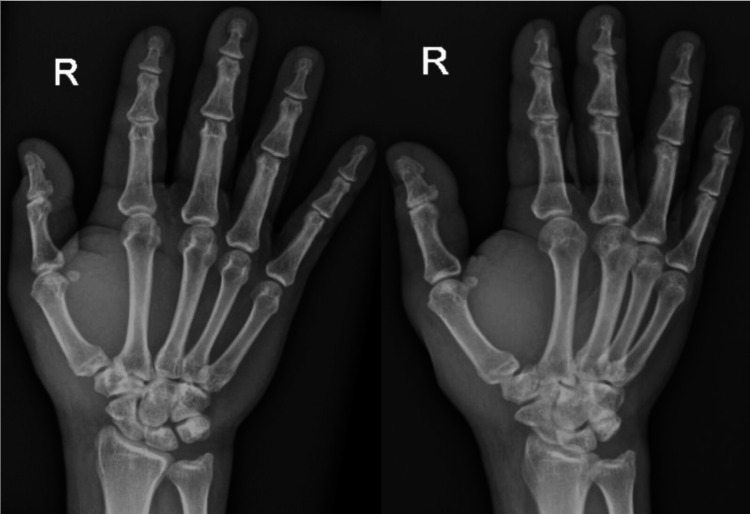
Radiograph of the patient’s right hand in anteroposterior (AP) and oblique views showing no presence of a foreign body.
